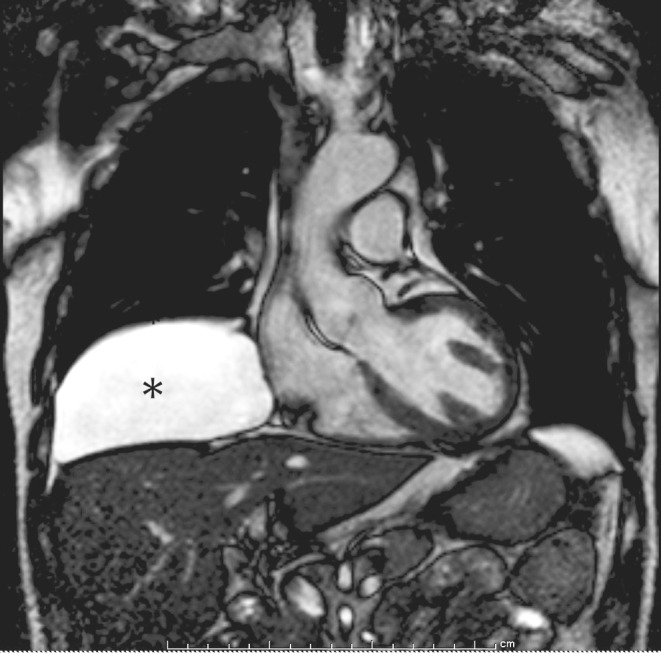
Cardiac magnetic resonance imaging showing a large mass outside the pericardium, with high signal intensity on T2-weighed imaging, most consistent with a pericardial cyst (*asterisk*).
